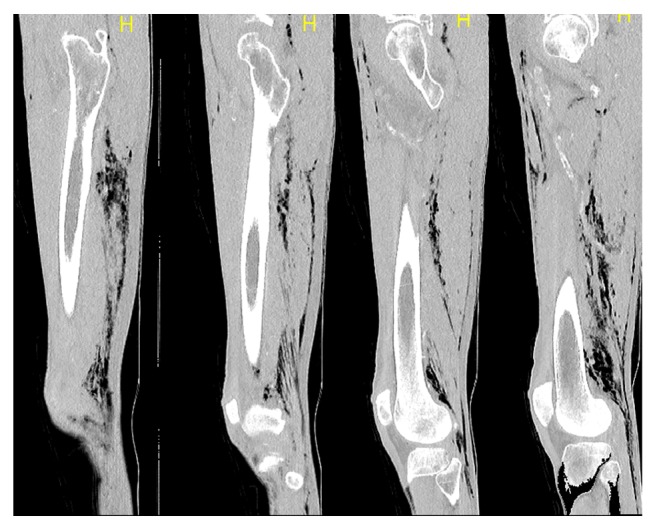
Sagittal sequence of the same CT scan.
